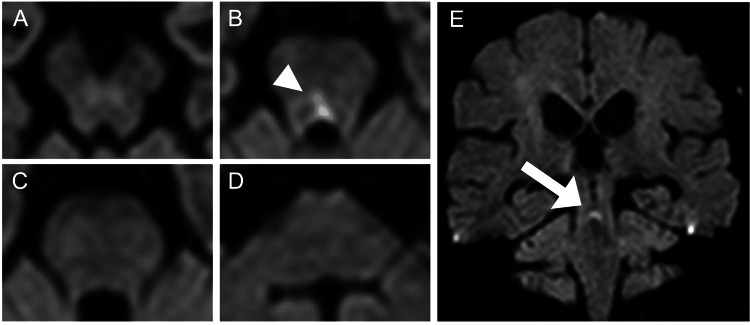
Brain MRI Axial (A: Midbrain, B: Upper pons, C: Middle pons, D: Lower pons) and coronal (E) diffusion-weighted image showing acute pontine tegmental infarction.
